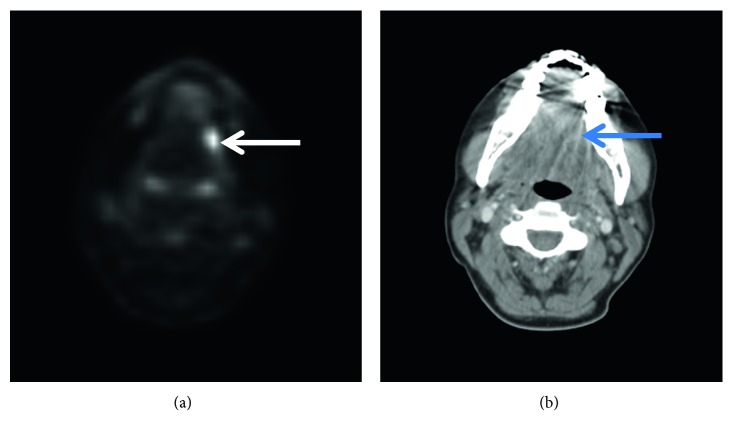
Initial axial PET scan (a) and CT scan (b) demonstrating hyperactivity along the left lateral tongue ((a) white arrow; (b) blue arrow).
